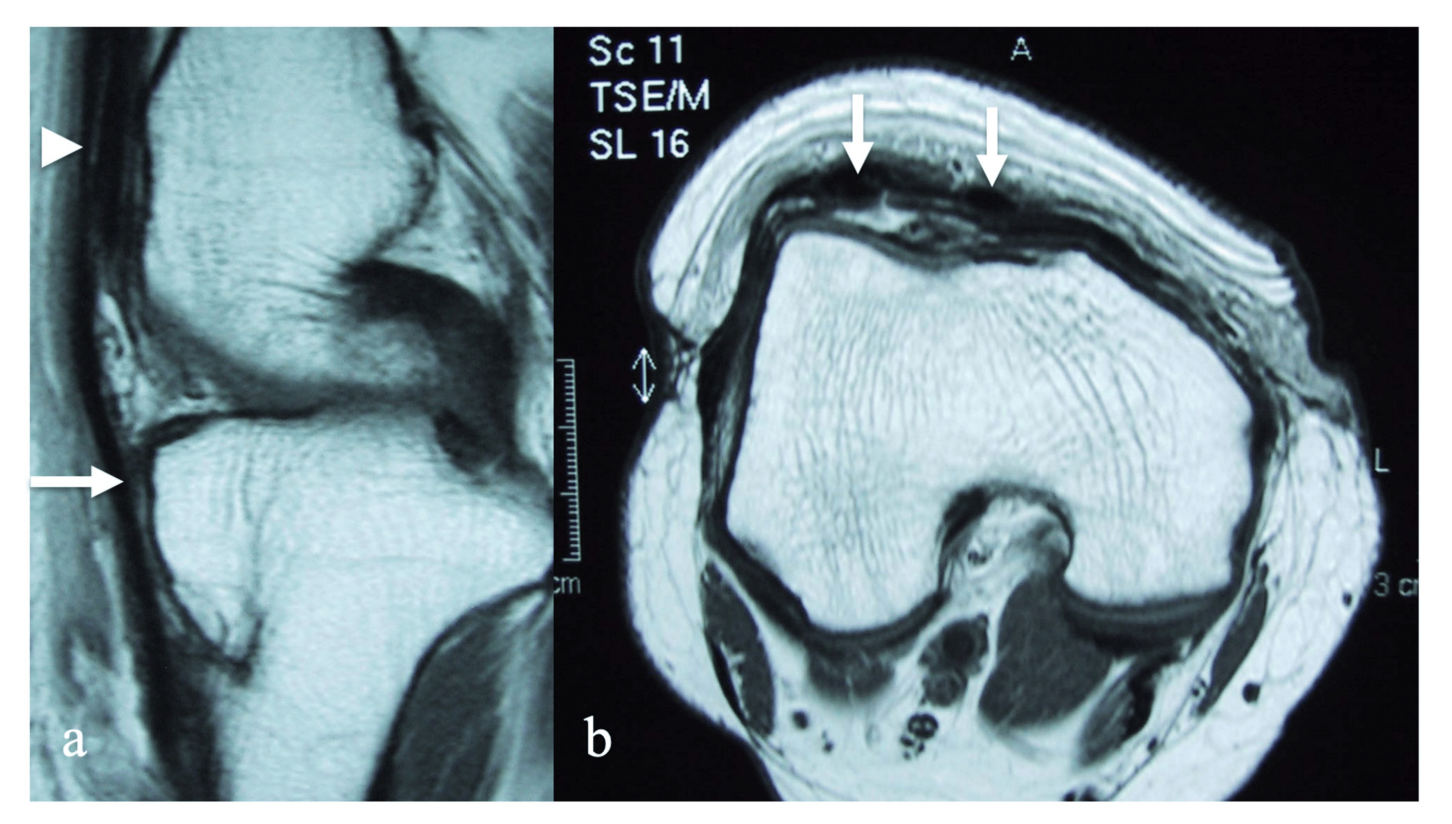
MRI of the right knee 1 year after surgery. A: Sagittal view
